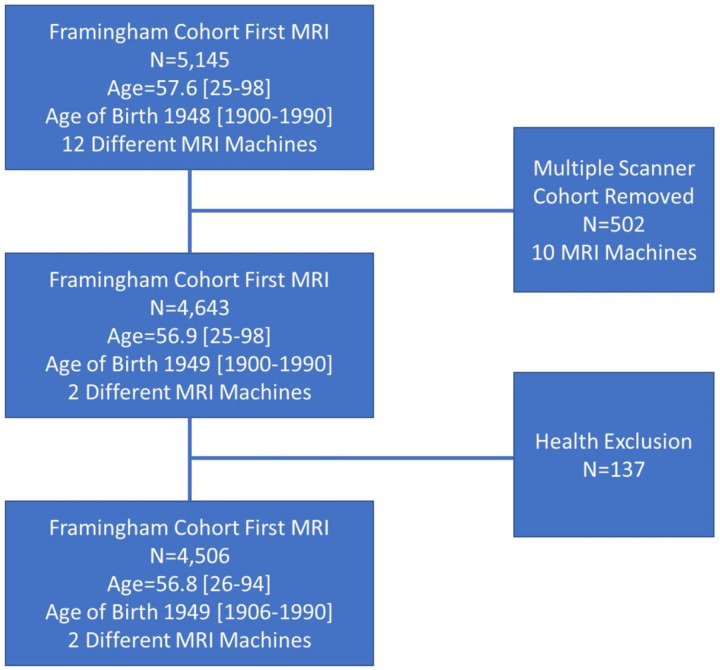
Sample Selection Figure 1 summarizes subject selection with mean age and standard deviation. All individuals were included at the time of the first MRI. Subjects were imaged on a total of 12 different MR machines, but greater than 90% of participants were imaged on only 2 different scanners leading to removal of 502 participants from study due to imaging on alternative scanners. Finally, individuals with stroke, dementia of other neurological disorder were excluded resulting a study cohort of 4506 individuals.
